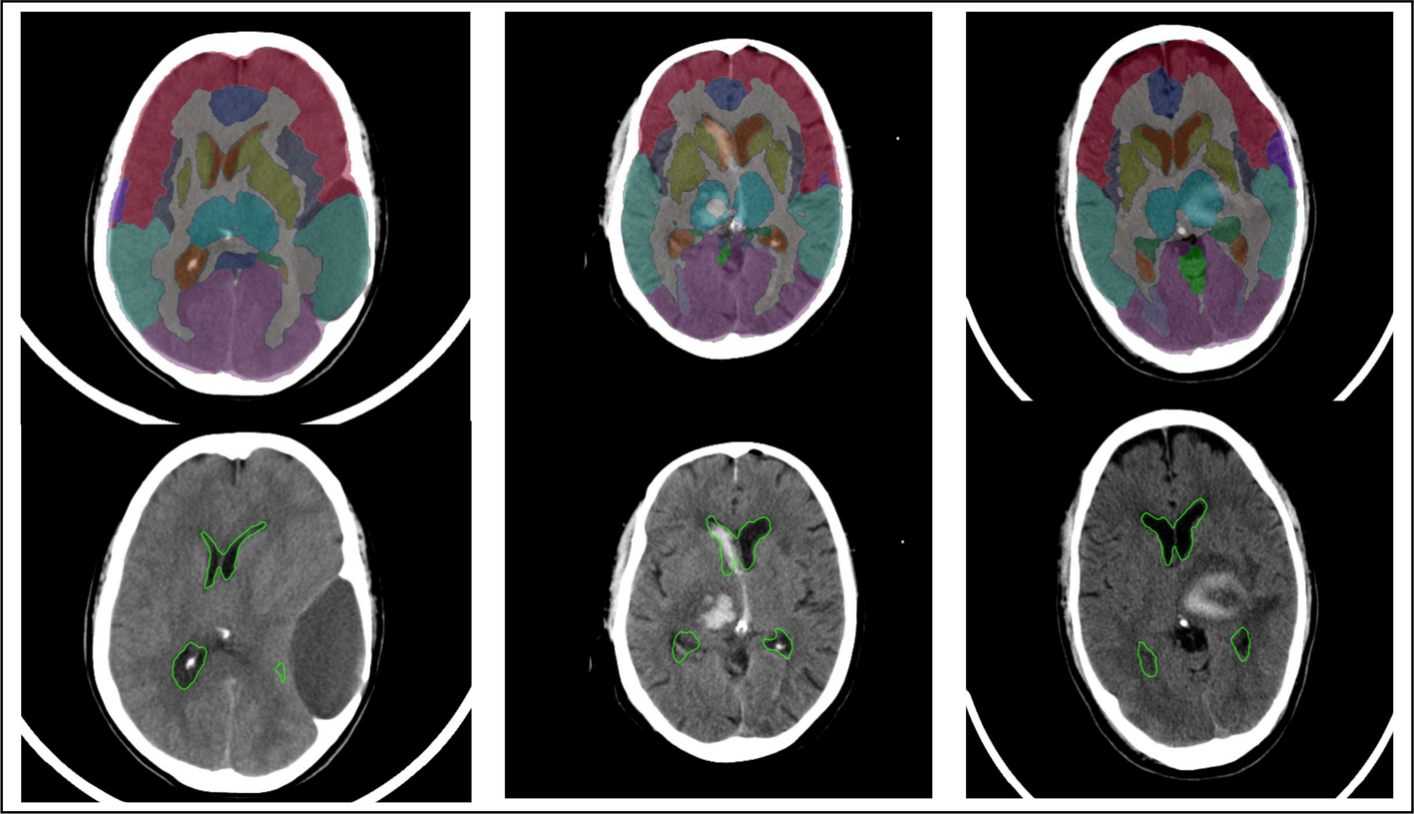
Three exemplary CT scans with pathologies and atlas labels that were propagated with our approach. First row: all atlas labels, second row: only label for lateral ventricles
